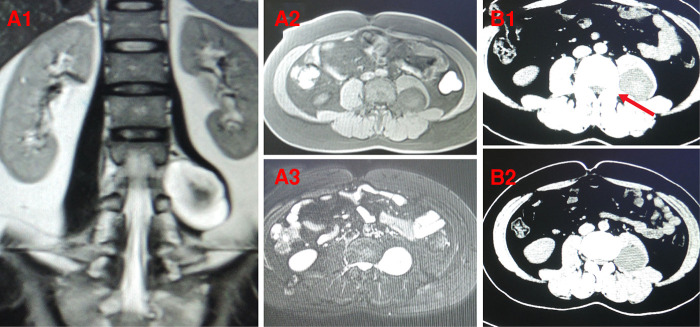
Preoperative MRI and CT showed lumbar dumbbell-shaped tumor of a 34-year-old male patient (case 5) (A,B). (A1) coronal MRI; (A2, A3) axial MRI; (B1, B2) axial CT. The pedicles of adjacent vertebra to lumbar dumbbell-shaped tumor (L3/L4) were intact. The red arrow indicates intact pedicle.
